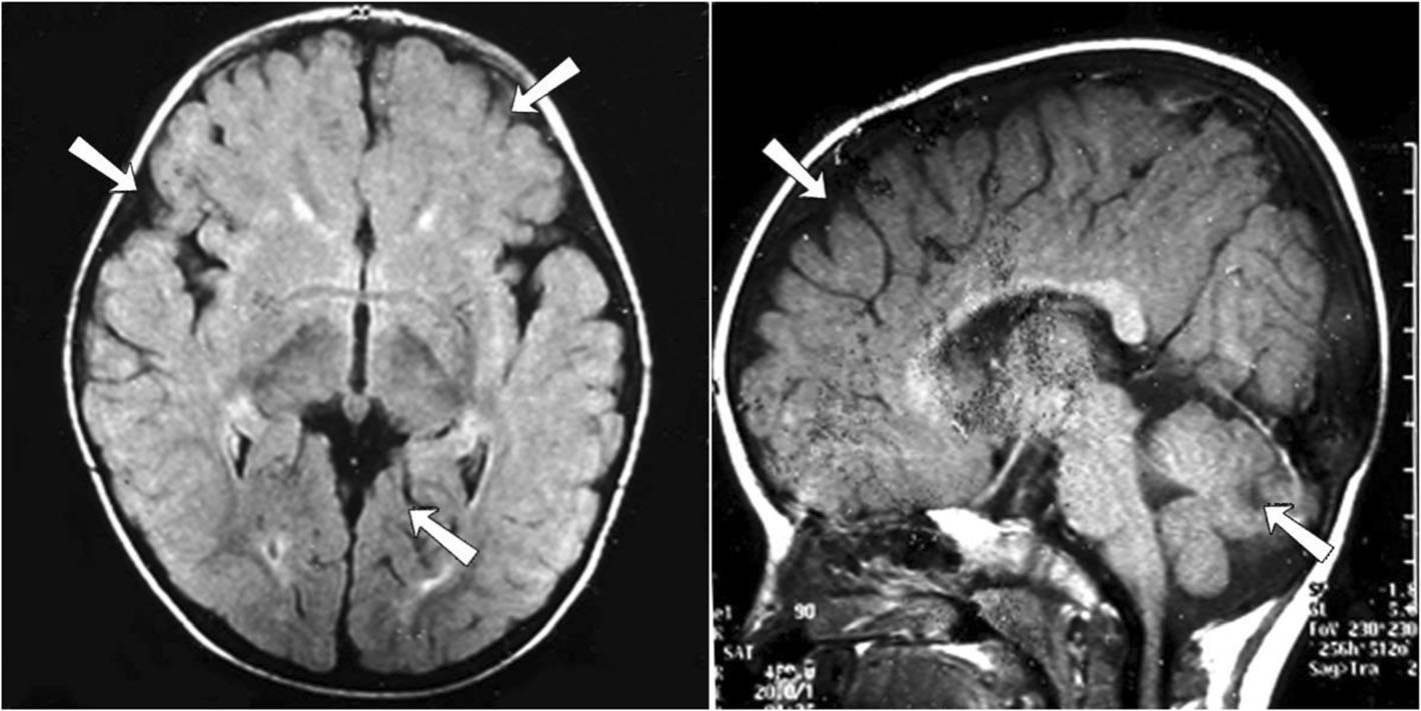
MRI brain performed at 10 years of age revealing cerebellar especially vermis hypoplasia, cerebral atrophy and white matter changes
